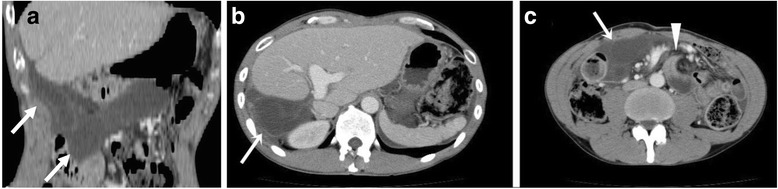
Enhanced abdominal CT scan on an arrival a Coronal plane. b, c Transverse plane. a–c CT demonstrated dilated, fluid-filled small bowel loops with poor enhancement of the bowel wall. Arrows indicate a markedly dilated Roux limb. c. The triangle arrow indicates a “whirl” appearance
